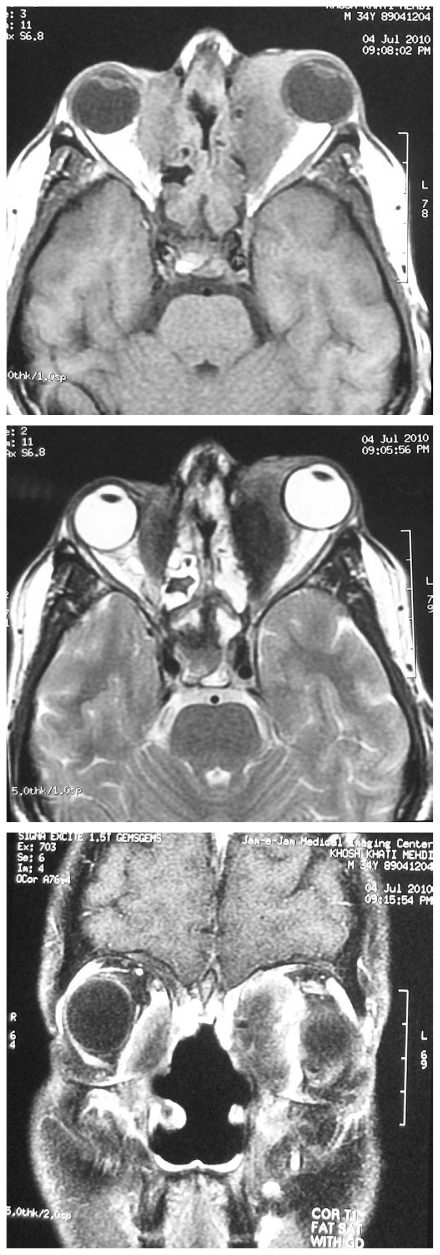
Orbital magnetic resonance imaging shows heterogenous bilateral orbital mass lesions adjacent to the medial walls. The lesions have moderate intensity, similar to muscle on axial T_1_-weighted (top) and low intensity on axial T_2_-weighted images (middle). On coronal fat suppression T_1_-weighted view with contrast (bottom) the lesions show marked heterogenous enhancement. Diffuse mucosal thickening in the paranasal sinuses and absence of the nasal septum are seen in all images.
